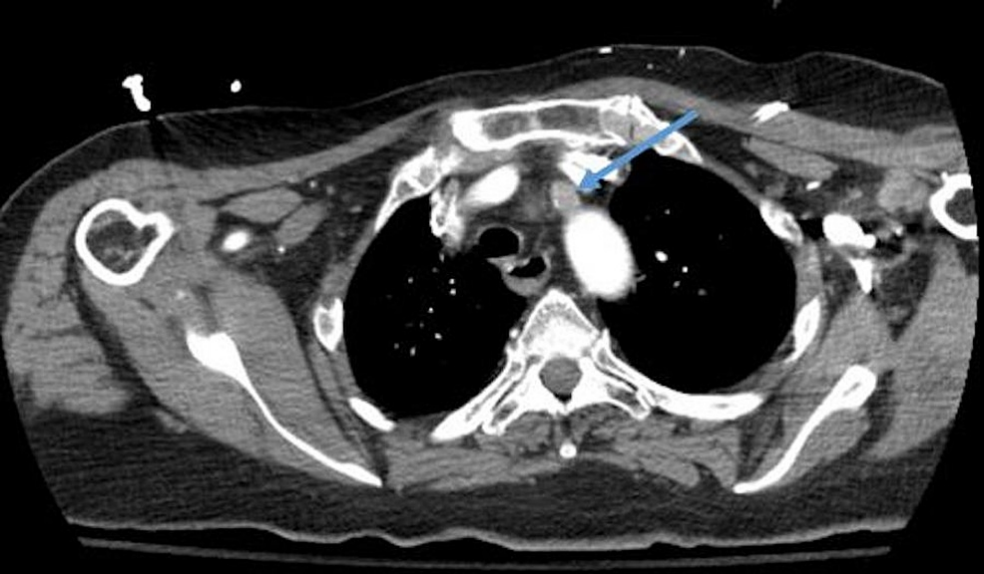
Computed tomography angiography (CTA) - blue arrow showing thrombus extending to left subclavian artery
